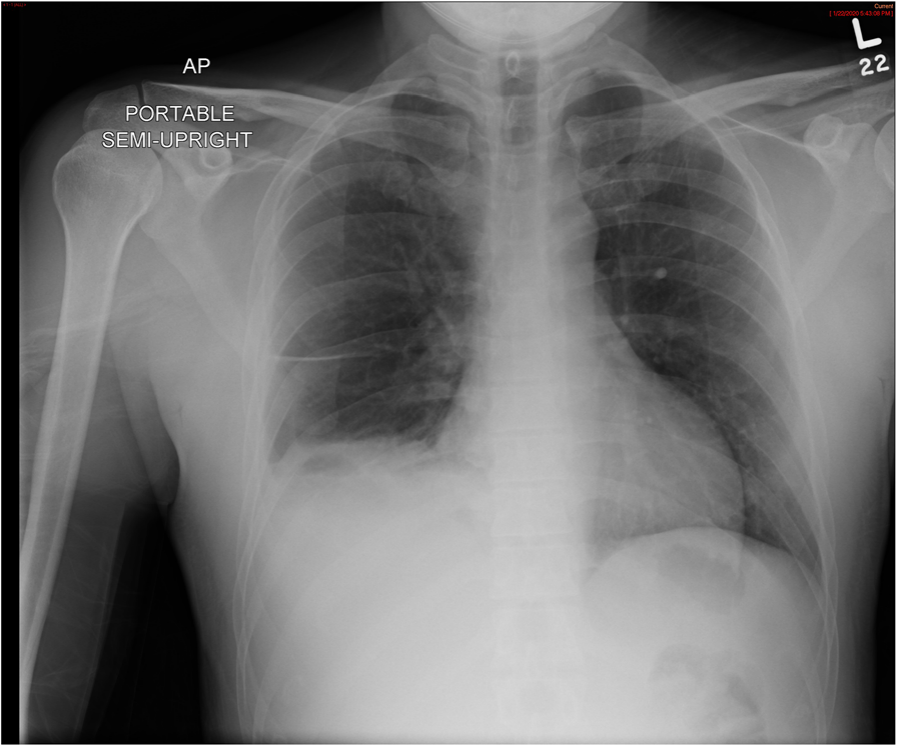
Chest radiograph of the patient showing a right pleural effusion
